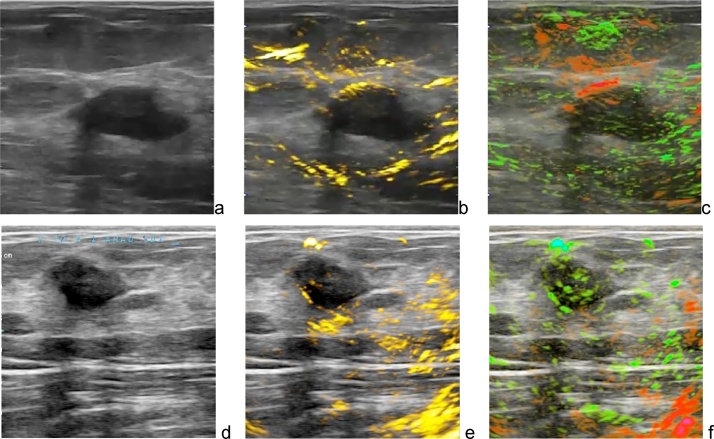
Two examples of OA/US images of benign fibroadenoma. Optoacoustic functional images invert the conclusion based on ultrasonic morphological image, downgrading BIRADS to 3. Images are 38 × 38 mm.
